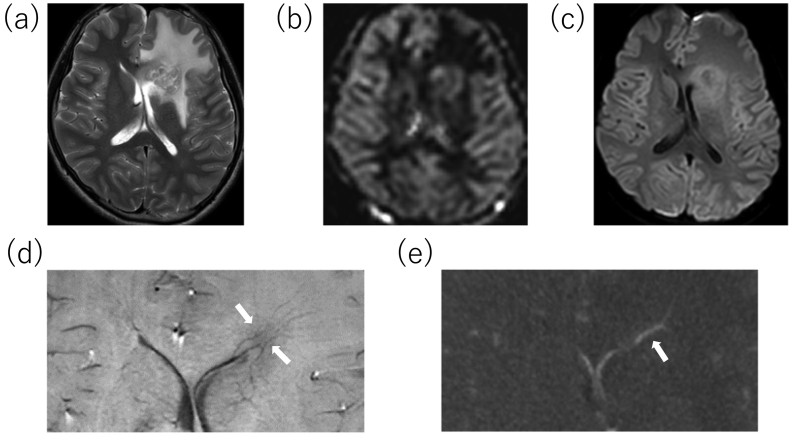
(a) On T2-weighted images, lesions appear hypointense and surrounded by extensive edema with no hypointense rim. (b) Arterial spin labeling image shows increased blood flow only in the merged area, not in the internal lesion. (c) Peripheral restriction around the lesion shown on a diffusion-weighted image. (d) Susceptibility-weighted image shows that veins passing through the lesion centers are visibly engorged (arrows). (e) Contrast-enhanced computed tomography image also reveals the engorged central vein (arrow).
